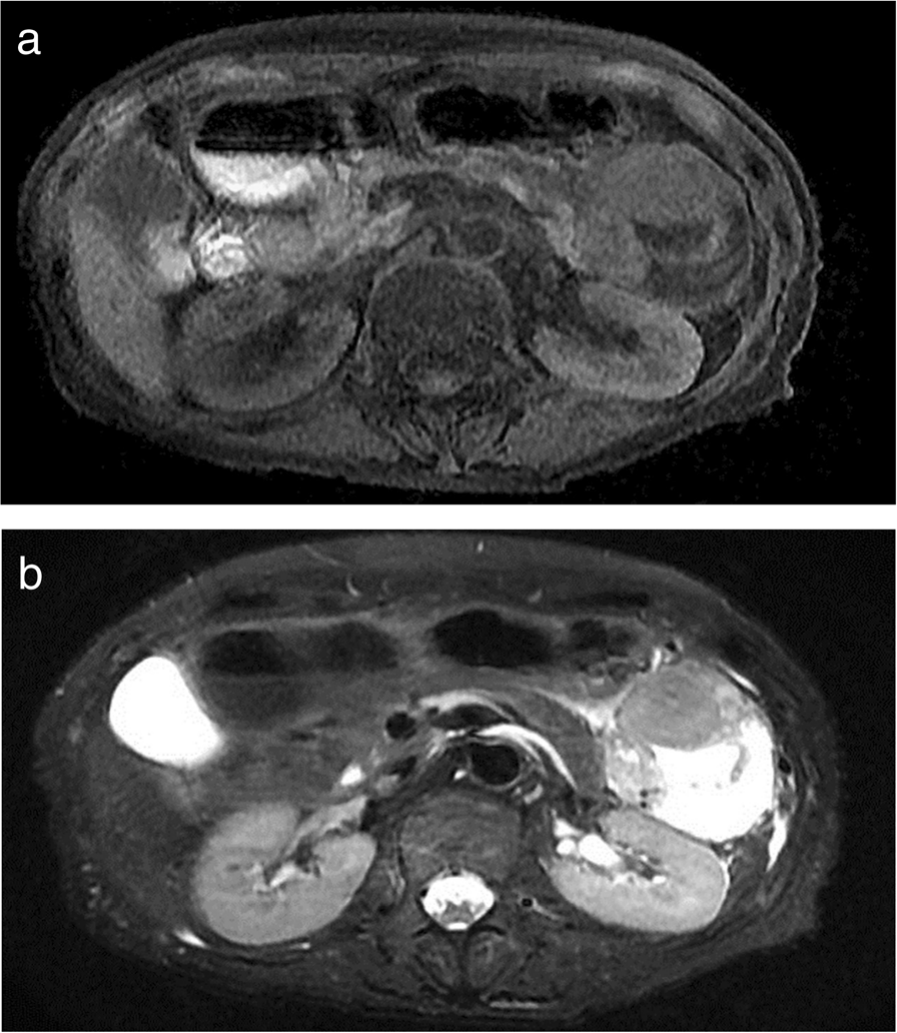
Magnetic resonance imaging (MRI) findings. a Solid components were hypointense to the pancreatic parenchyma on T1-weighted imaging. b On T2-weighted imaging, solid components were hyperintense. A large non-enhancing portion on the CT scan showed bright signal intensity, indicating cystic change or necrosis
